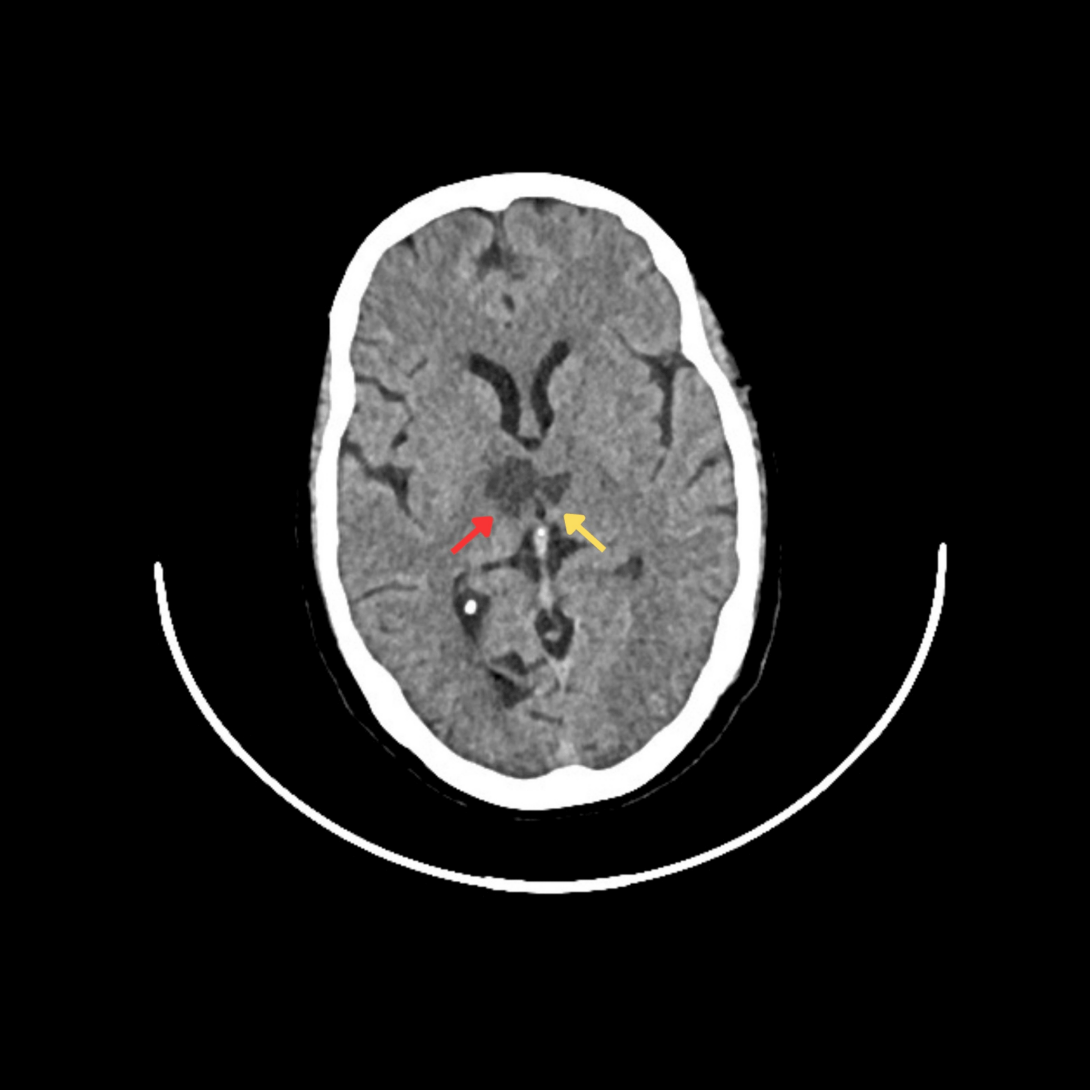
Computed tomography (CT) scan of the brain CT brain axial view displaying distinct area of hypoattenuation in the bilateral paramedial thalamus(right thalamus, red arrow; left thalamus, yellow arrow) advancing into bilateral midbrain resulting in impingement of third ventricle in its posterior aspect.
